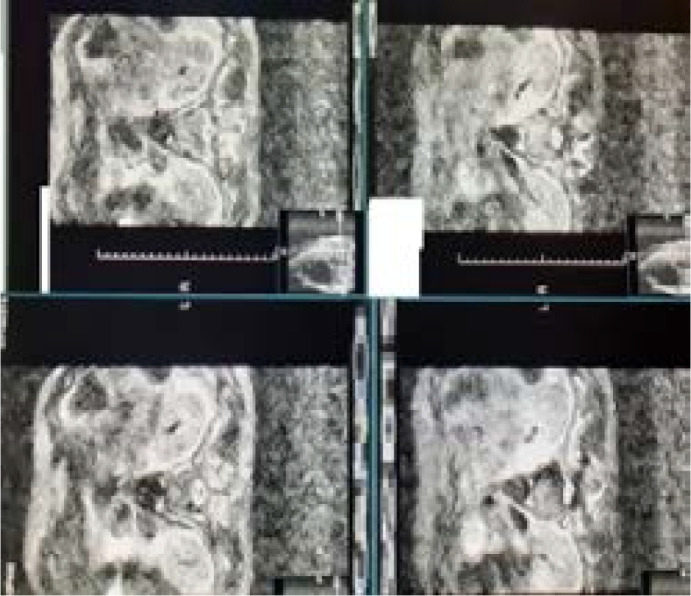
Motion induced artifact seen on lumbar spine MRI.
